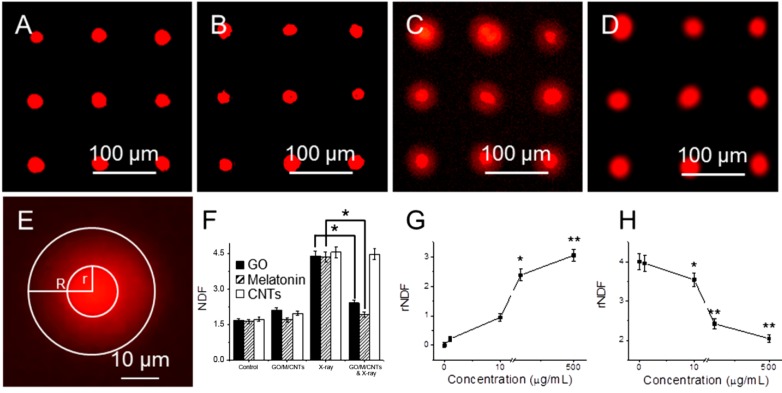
Genotoxicity of cells treated with GO and X-ray irradiation with halo assay. Fluorescent images of arrayed cells (A); cells treated with 10 µg/mL GO (B); cells exposed to 1.25 Gy X-ray (C); and cells treated with GO and then exposed to 1.25 Gy X-ray (D); an enlarged image shows that halo and nucleus (E); the NDF values of cells after different treatment (F); the rNDF values of cells treated with different concentration of GO without (G) and with 1.25 Gy X-ray radiations (H). “*” (*p* < 0.05) and “” (*p* < 0.01) represent significant difference and extra significant difference, respectively.
